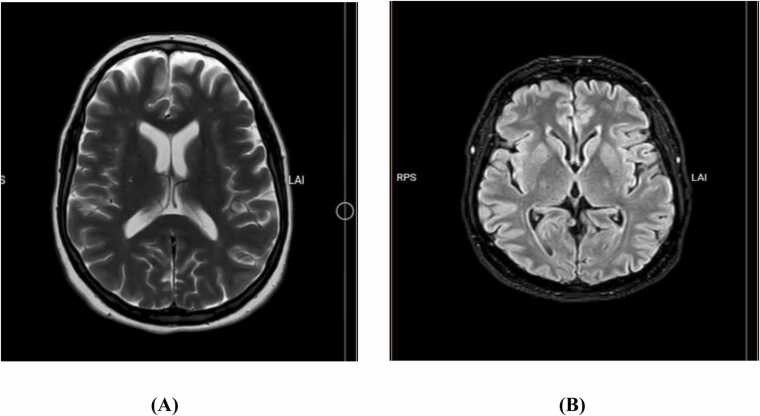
MRI in T2 (A) and FLAIR (B) sequences.
